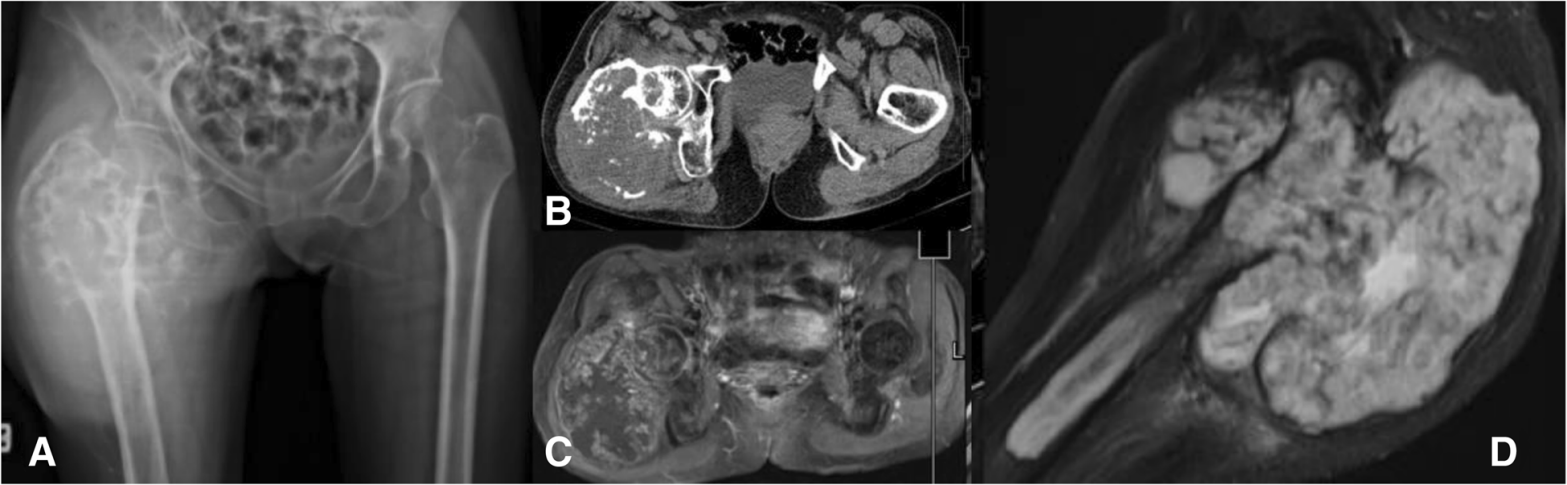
A 73-year-old female, with chondrosarcoma right proximal femur. a The film images of both hips showing large bony lesion with suspected chondroid matrix within the right proximal femur, (b) Axial CT scan revealing cortical breakthrough with soft tissue extension, (c) Axial MRI revealing large mass with heterogeneous enhancing lesion, (d) sagittal MRI revealing tumor margin extending into the mid-shaft of the femoral medullary canal
